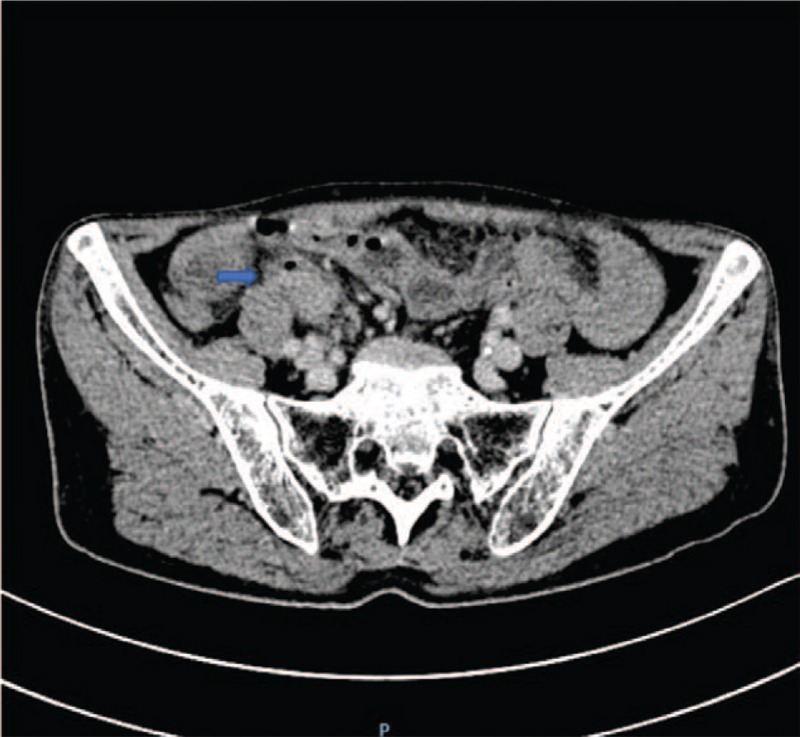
Abdominal computed tomography showed thickened bowel with enhancement (arrow indicates the abnormal bowel).
